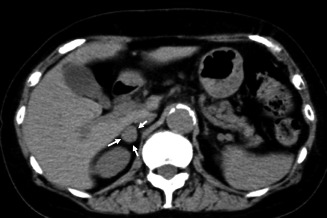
CT revealed a right adrenal tumor (white arrow).
